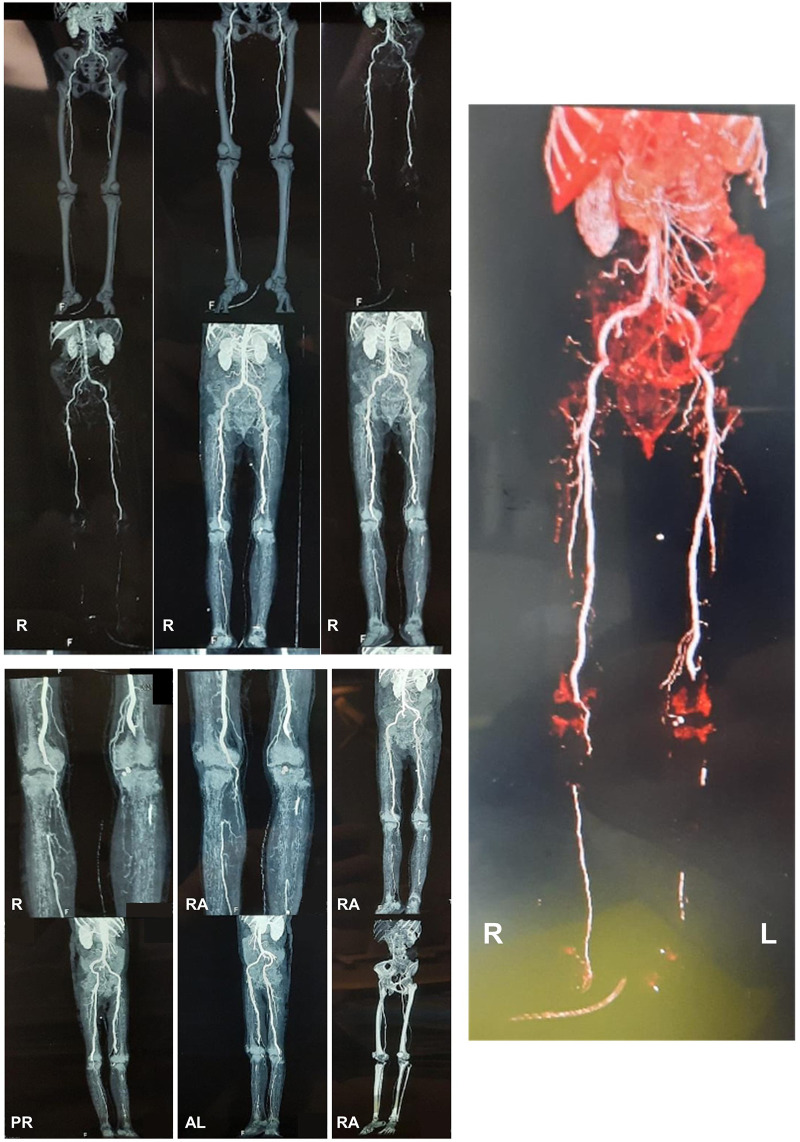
The computed tomography angiography (CTA) of the lower extremity. The CTA revealed an abdominal aortosclerosis with abnormalities on both limbs. Right limb: total occlusion due to 6 cm thrombus on right popliteal artery (from 1 cm above right femorotibial joint toward inferior), right anterior tibial artery received contrast flow from collateral arteries and no contrast flow was seen in right posterior tibial, peroneal and dorsalis pedis arteries. Left limb: total occlusion due to 12.7 cm thrombus on left popliteal artery (from 6 cm above left femorotibial joint toward inferior). No contrast flow was seen in left anterior tibial, posterior tibial, peroneal and dorsalis pedis arteries. (This figure has been edited in Microsoft PowerPoint 2016 to obscure patient’s data.)
